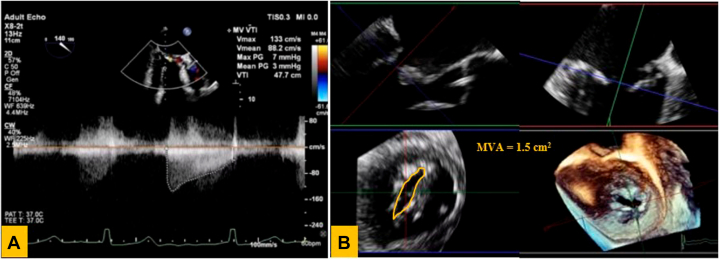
Postinterventional Transesophageal Echocardiography, Patient 2 (A) Reduction in the mean transmitral gradient to 3 mm Hg. (B) Increase in mitral valve area (MVA) to 1.5 cm^2^ as assessed by 3-dimensional planimetry.
